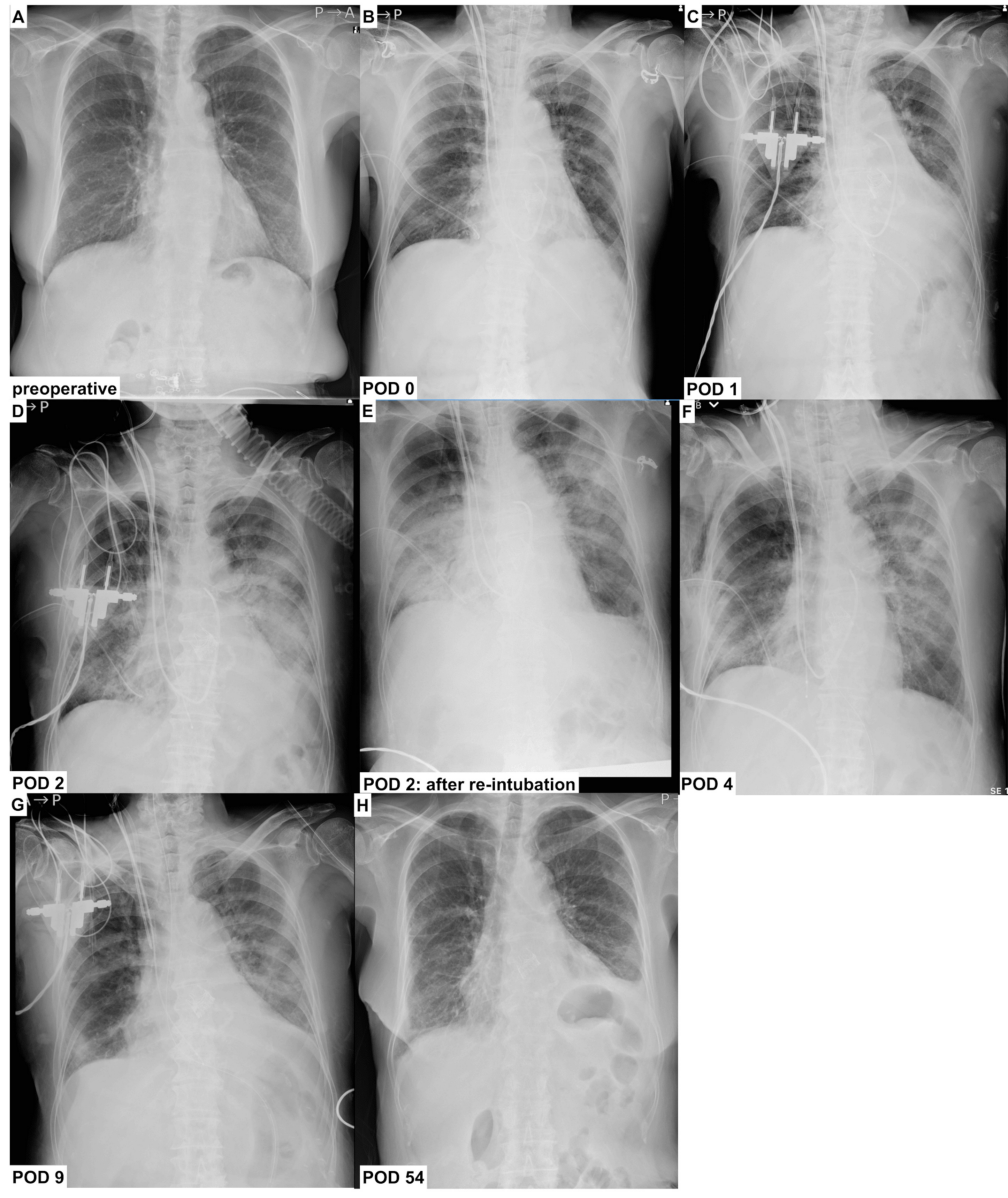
Changes in chest radiography through disease course A: preoperative X-ray; B: POD 0; C: POD 1; D: POD 2; E: POD 2, after re-intubation; F: POD 4; G: POD 9; H: POD 54 POD: postoperative day
